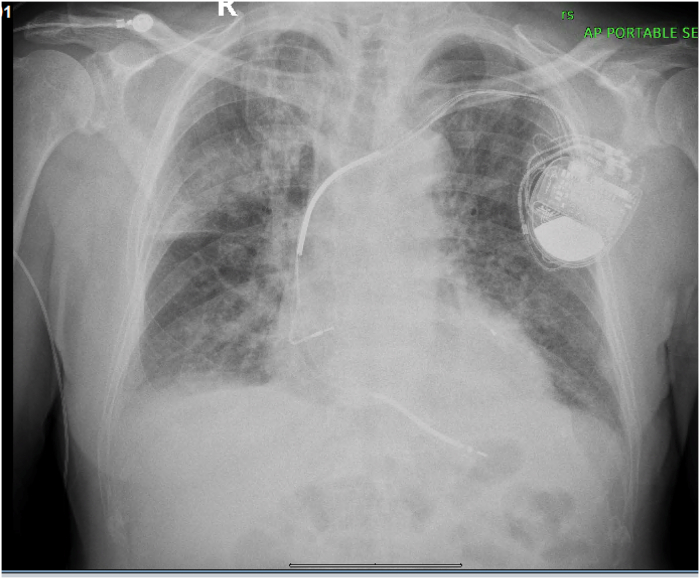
Portable Anteroposterior Chest Radiograph Showing Bilateral Alveolar-Interstitial Infiltrates Predominantly in the Perihilar and Lower Lung Zones, Consistent With Acute Pulmonary Edema
